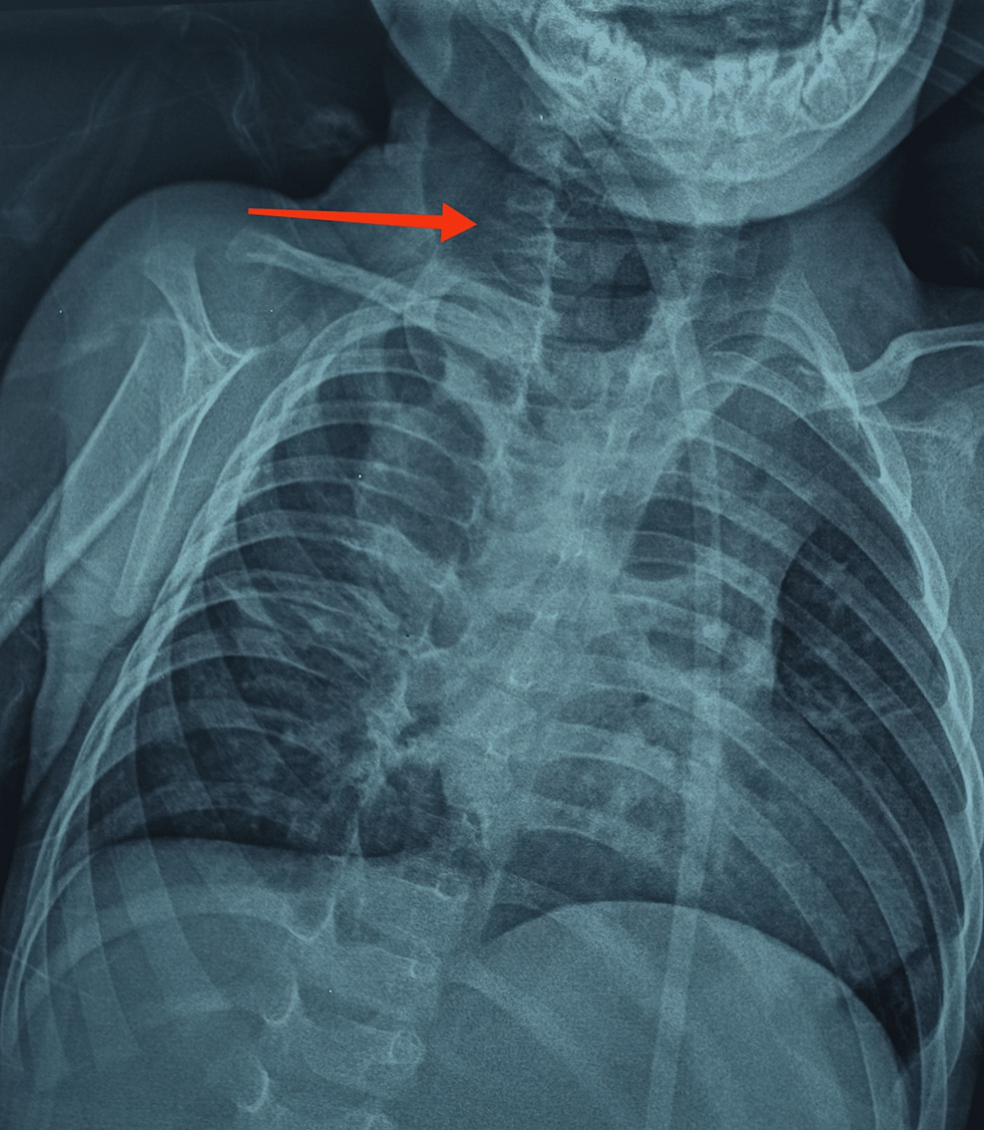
Cervical emphysema bubble on chest X-ray
